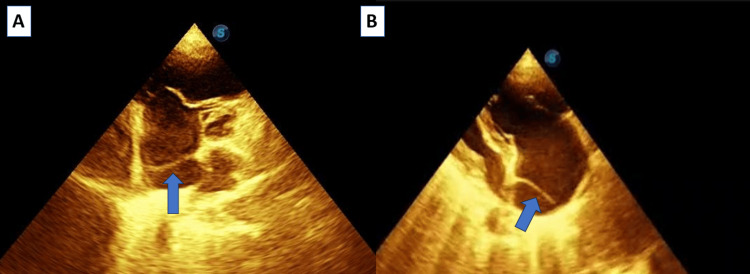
(A) TTE at the level of the great vessel and (B) at apical four-chamber views showing a membrane at the right atrium, which separates the right atrium into two structures TTE: transthoracic echocardiography
